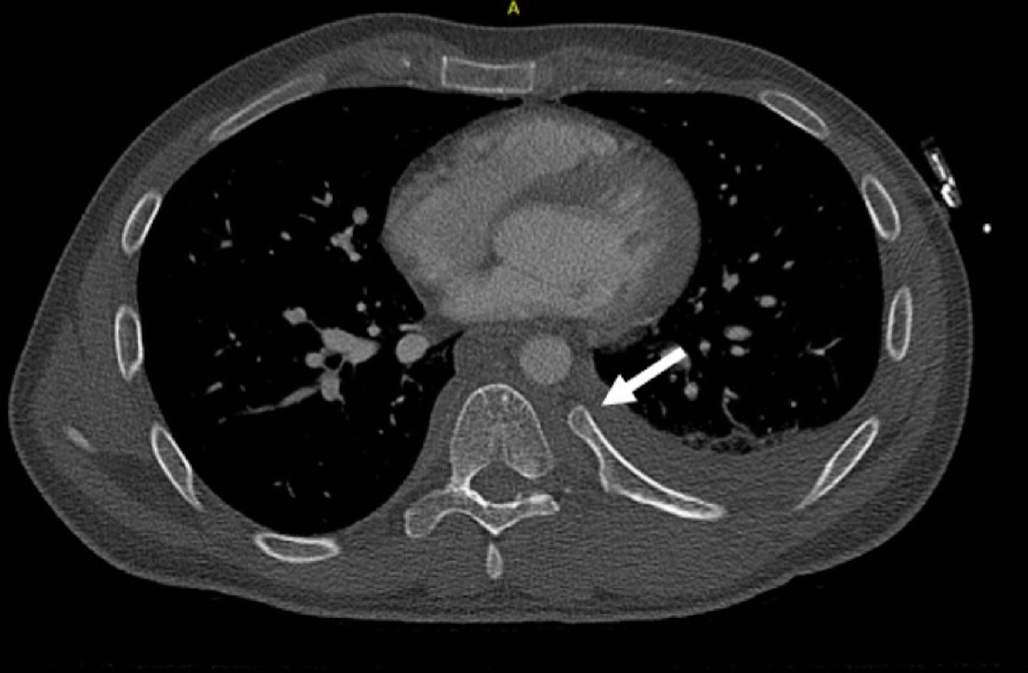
Transverse CT-scan made during the initial trauma assessment. The arrow shows the costovertebral dislocation of the left seventh rib.
